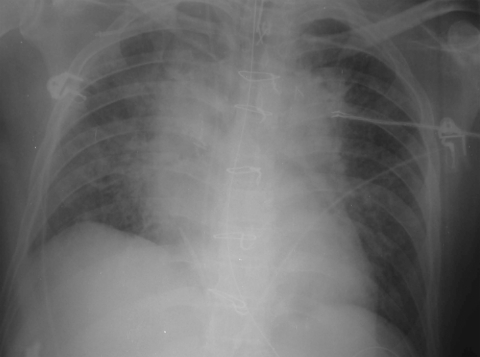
Chest radiograph of patient no. 5, who had community-acquired pneumonia associated with *Tropheryma whipplei*.
